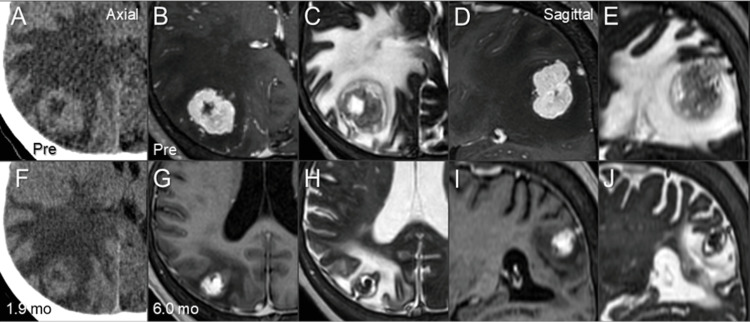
Computed tomography and magnetic resonance images before and after stereotactic radiosurgery. The images show axial images (A-C, F-H); sagittal images (D, E, I, J); computed tomography images (A, F); CE-T1-WI (B, D, G, I); T2-WI (C, E, H, J); before SRS (A-E); at 1.9 months after SRS (F); and at 6.0 months (G-J). (A-J) All images were co-registered and are shown in the same magnification (A-J) and coordinates (A-F). (F) At 1.9 months after SRS, considerable shrinkage of the BM was observed along with modest alleviation of the perilesional edema and mass effect. (G-J) At 6.0 months, marked shrinkage of the BM and mitigation of the peritumoral edema were observed along with extrication from the mass effect. CE: contrast-enhanced; WI: weighted image; SRS: stereotactic radiosurgery; BM: brain metastasis
